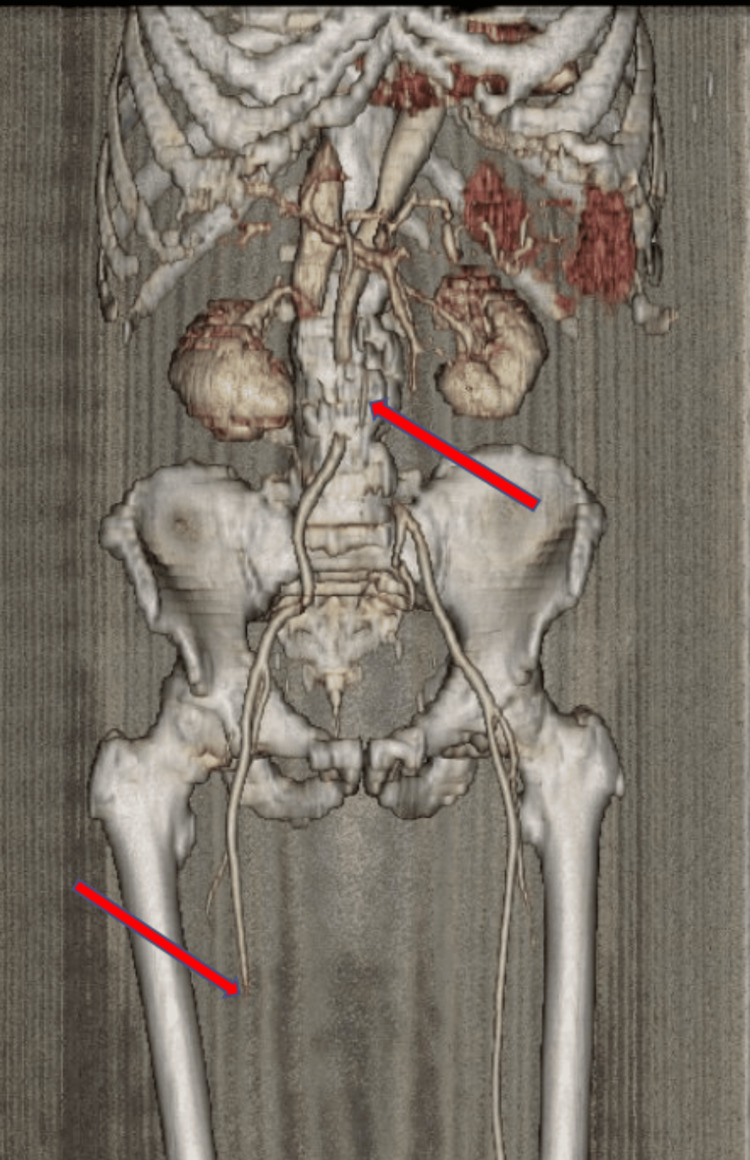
3D reconstructed CT angiography of the aorto-iliac and femoral arteries. Demonstrates extensive thrombus extending from the abdominal aorta into the bilateral iliac arteries, with poor distal runoff and complete occlusion of the right superficial femoral artery (red arrows). 3D: three dimensional, CT: computed tomography
